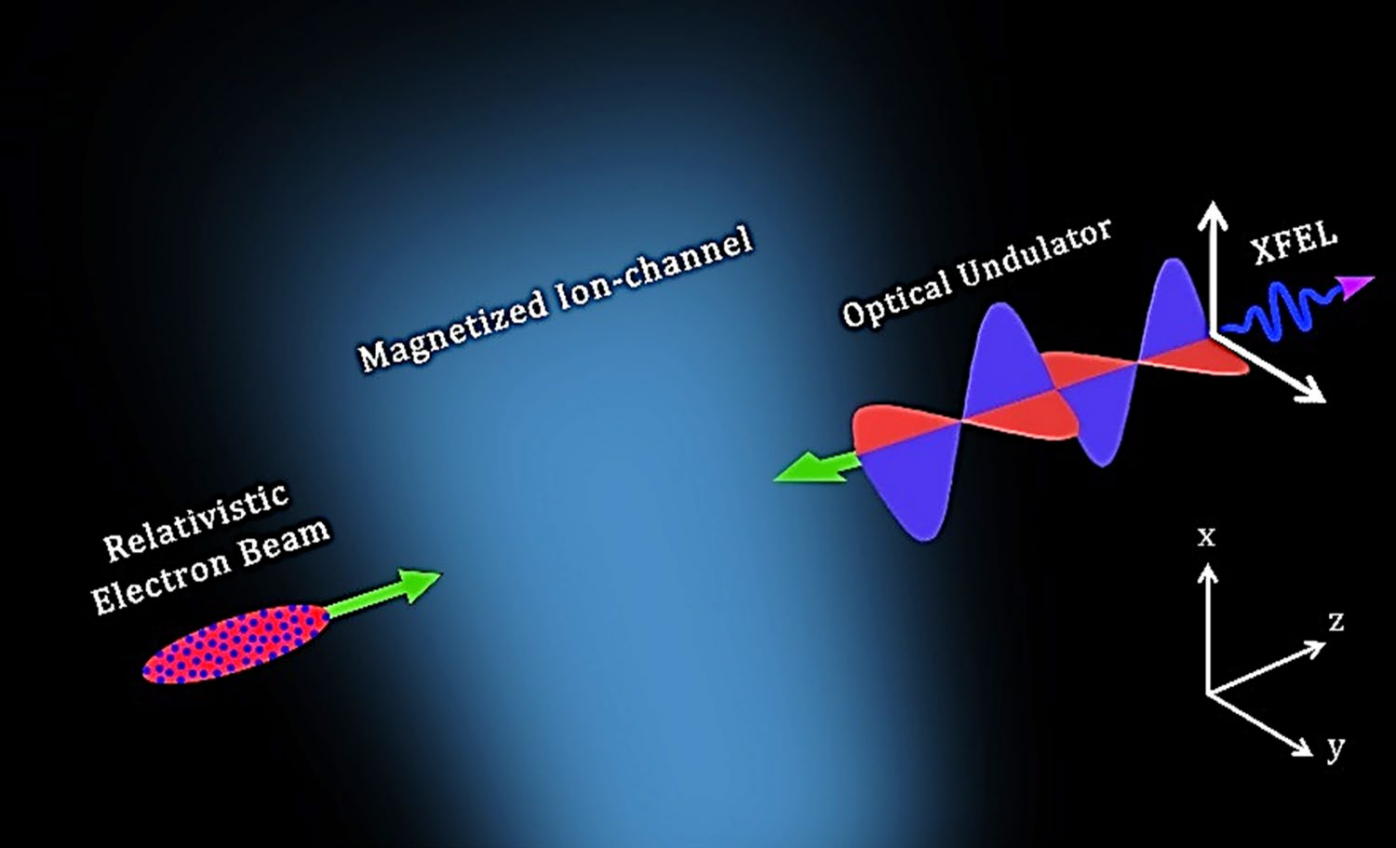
An X-ray FEL scheme based on an optical undulator in the presence of a magnetized ion-channel background.
